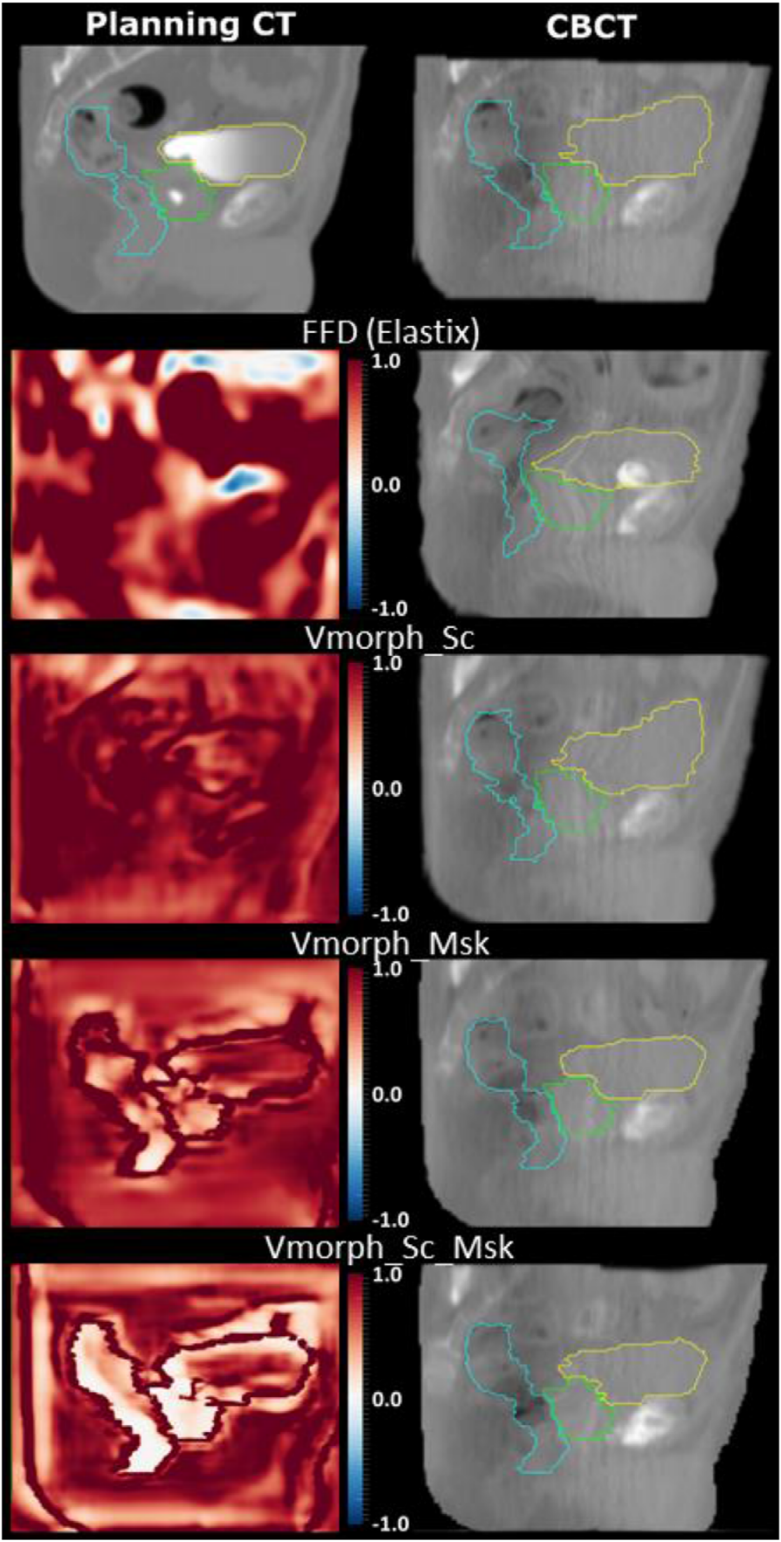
Qualitative evaluation of deformations per method for a median case in terms of DSC. The first row contains the planning and CBCT images. The second to fourth rows contain the Jacobian determinant and deformed CBCT images. The green, yellow, and light blue colors represent the prostate, bladder, and rectum, respectively. The image intensity parameters were 2000 and 0 for the window and level, respectively. The determinant of Jacobian intensity parameters were 2 and 0 for the window and level, respectively.
